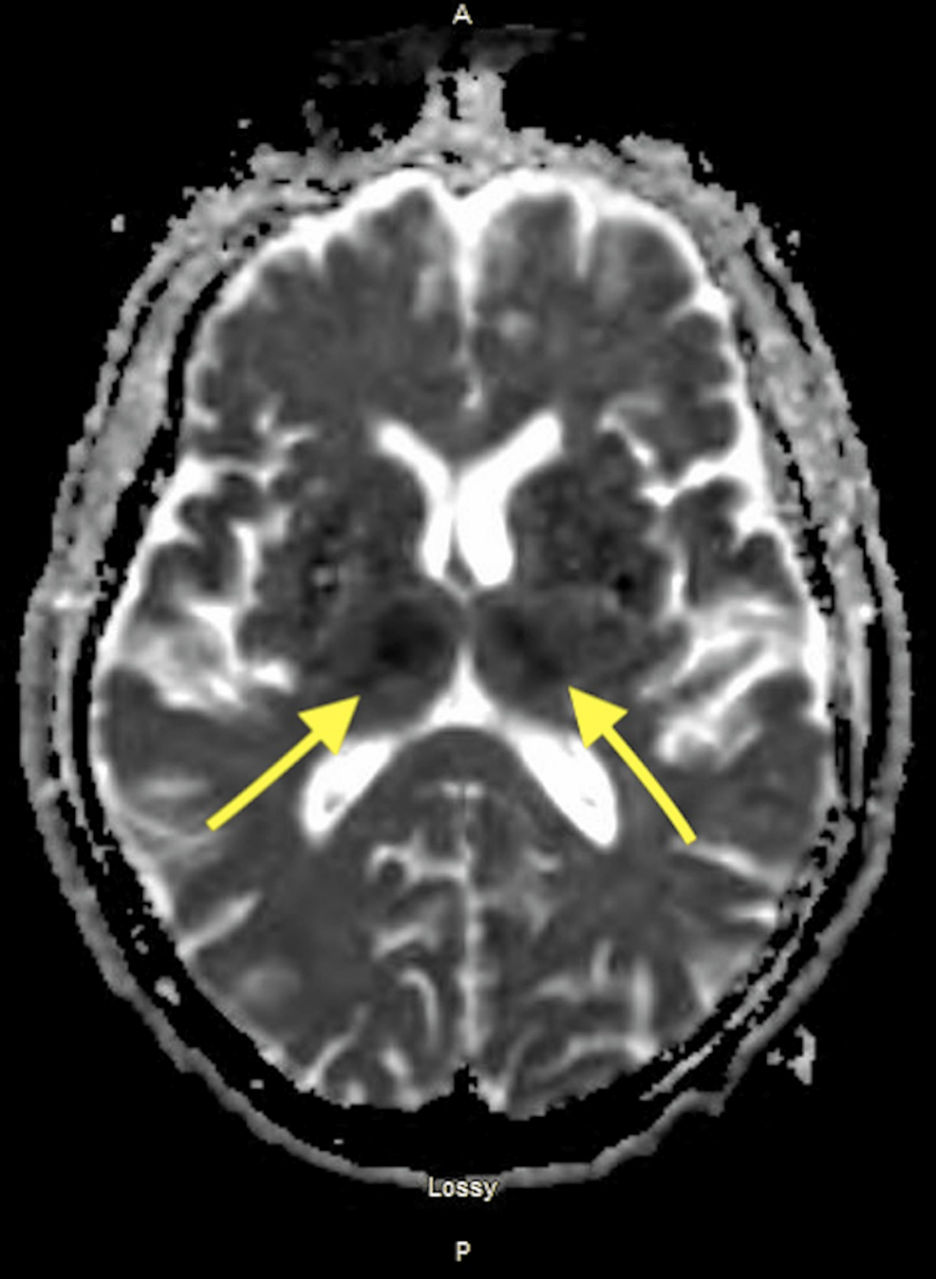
MRI of the brain without contrast demonstrating a decreased signal within bilateral thalami on ADC sequence reflecting the artery of Percheron infarct Decreased signal seen within bilateral thalami (arrows) ADC: apparent diffusion coefficient
